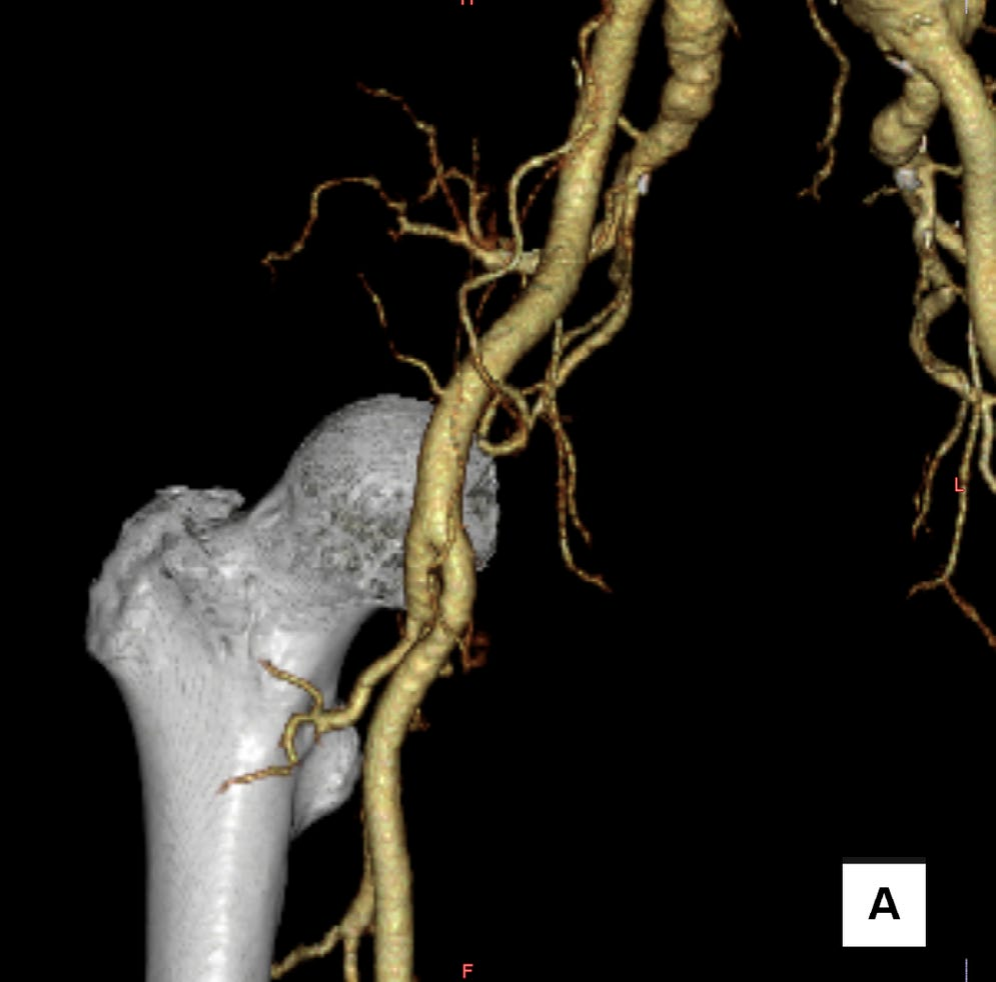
A three‐dimensional reconstruction image of the preoperative contrast‐enhanced computed tomography scan near the puncture site of the right femoral artery. The letter A denotes that the three‐dimensional reconstruction is shown from an anterior frontal view.
